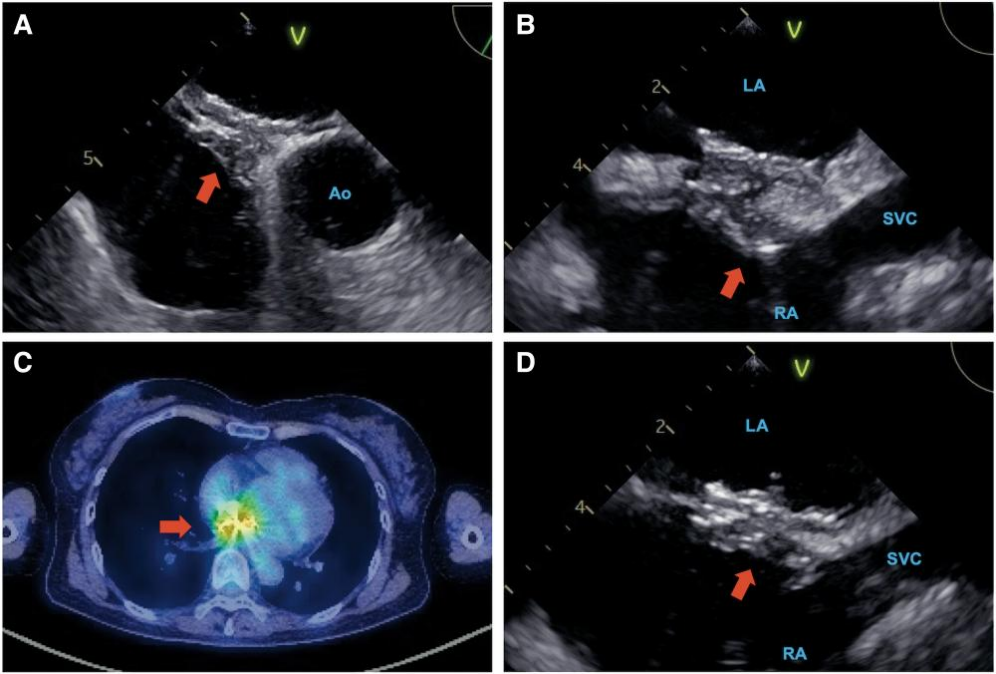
(*A* and *B*): (*A*) Mid-oesophageal aortic valve short-axis view, (*B*) mid-oesophageal bicaval view. The first transoesophageal echocardiography shows marked thickening of the atrial septal wall at the site of GORE CARDIOFORM septal occluder (GSO) implantation (arrow). (*C*) An ^18^F-fluorodeoxyglucose (FDG) positron emission tomography shows a high level of ^18^F-fluorodeoxyglucose accumulation at the site of the GORE CARDIOFORM septal occluder implanted in the atrial septum (arrow). (*D*) Mid-oesophageal bicaval view. The second transoesophageal echocardiography shows a remission of the atrial septal wall thickening at the site of the GORE CARDIOFORM septal occluder implantation (arrow). Ao, aorta; LA, left atrium; RA, right atrium; SVC, superior vena cava.
